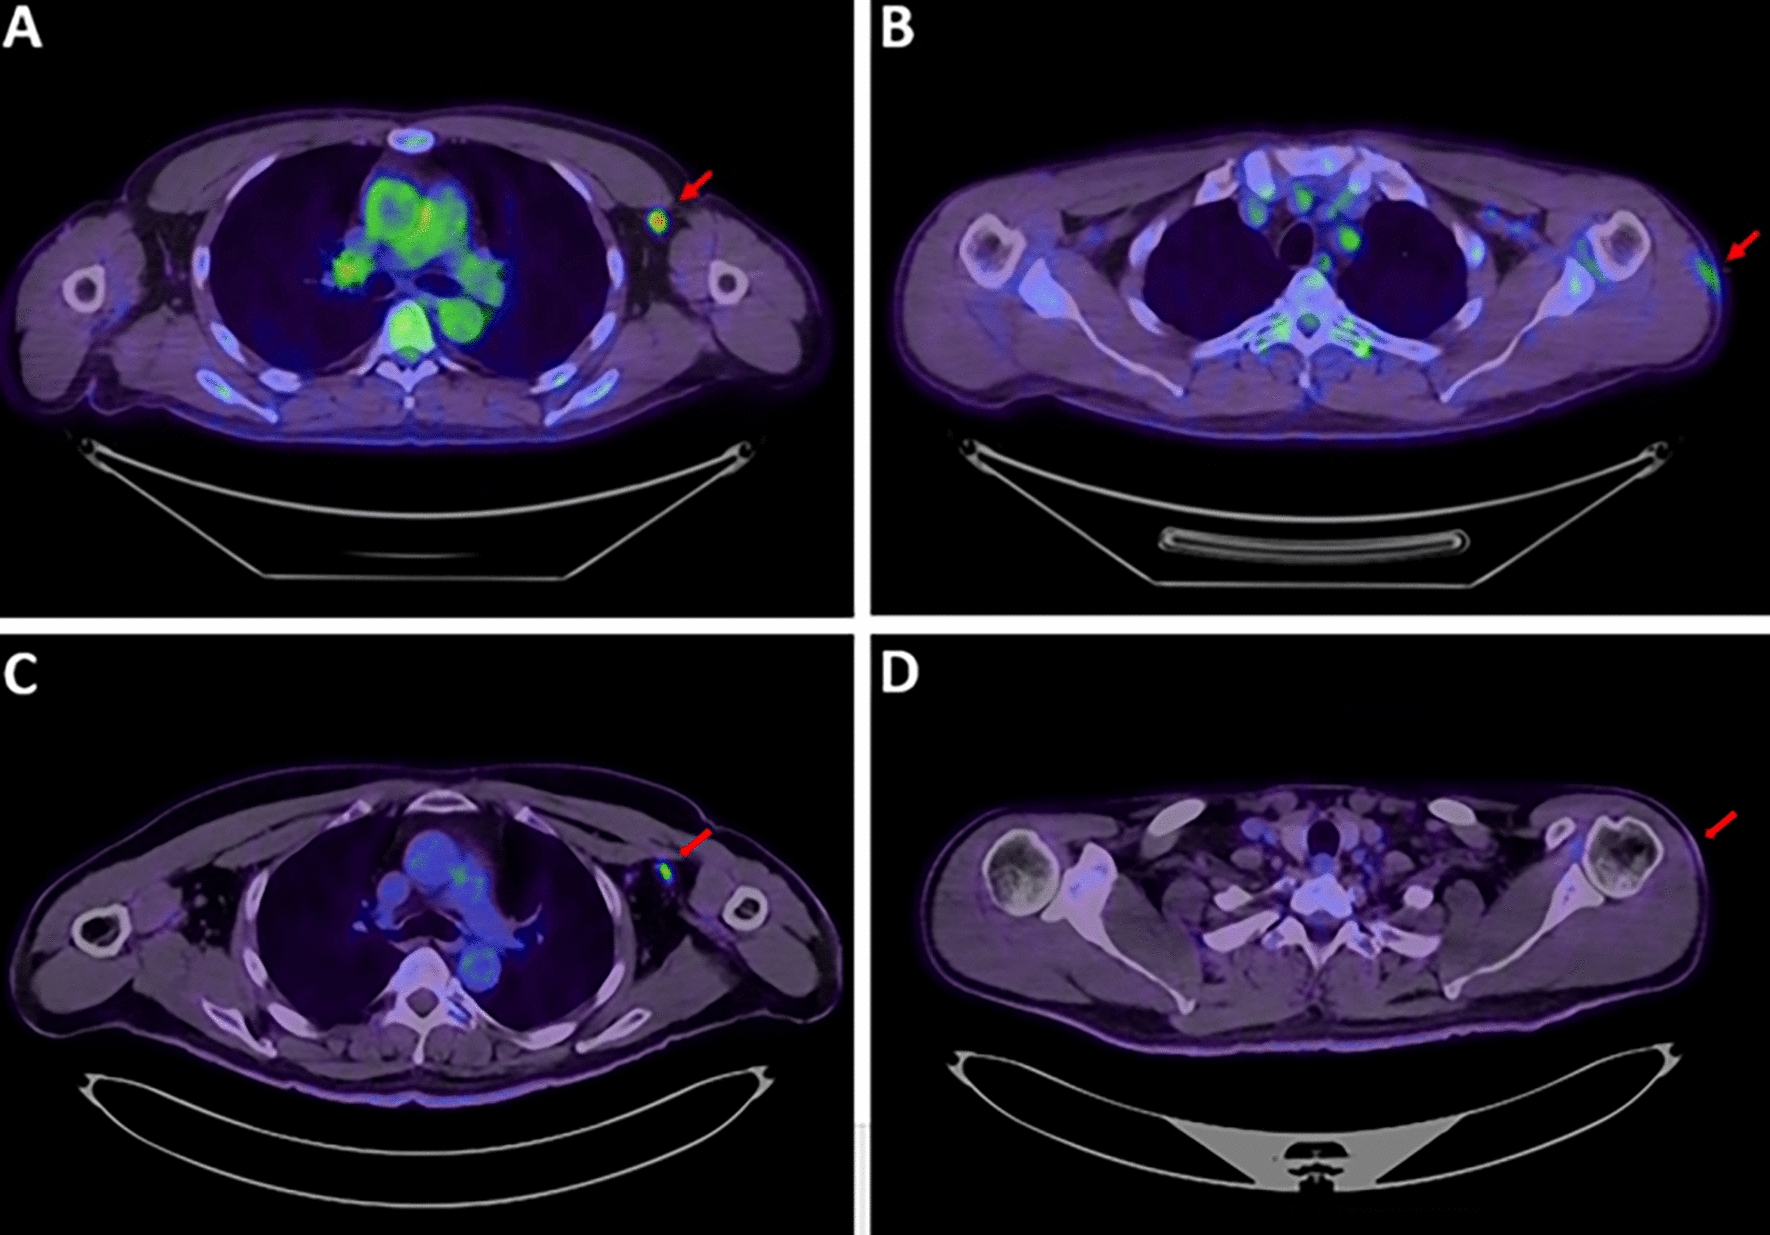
FDG PET/CT after COVID-19 vaccination. A and B A 42-year-old men underwent FDG-PET/CT for colon cancer staging. He received the first dose of the Oxford-AstraZeneca vaccine in the left arm 6 days before the scan. The SUVmax of HLN was 4.27, and the SUVmax of DL was 1.91. C and D A 54-year-old men underwent FDG-PET/CT for evaluation of recurrent colon cancer. He had his first dose of Moderna vaccine in the left arm 42 days before the scan. The SUVmax of HLN was 4.48, and the DL showed no hypermetabolism with SUVmax equal to 0.77. FDG PET/CT, ^18^F-fluorodeoxyglucose positron emission tomography/computed tomography; COVID-19, coronavirus disease 2019; HLN, hypermetabolic lymphadenopathy; DL, deltoid muscle uptake; SUVmax, maximal standard uptake value
